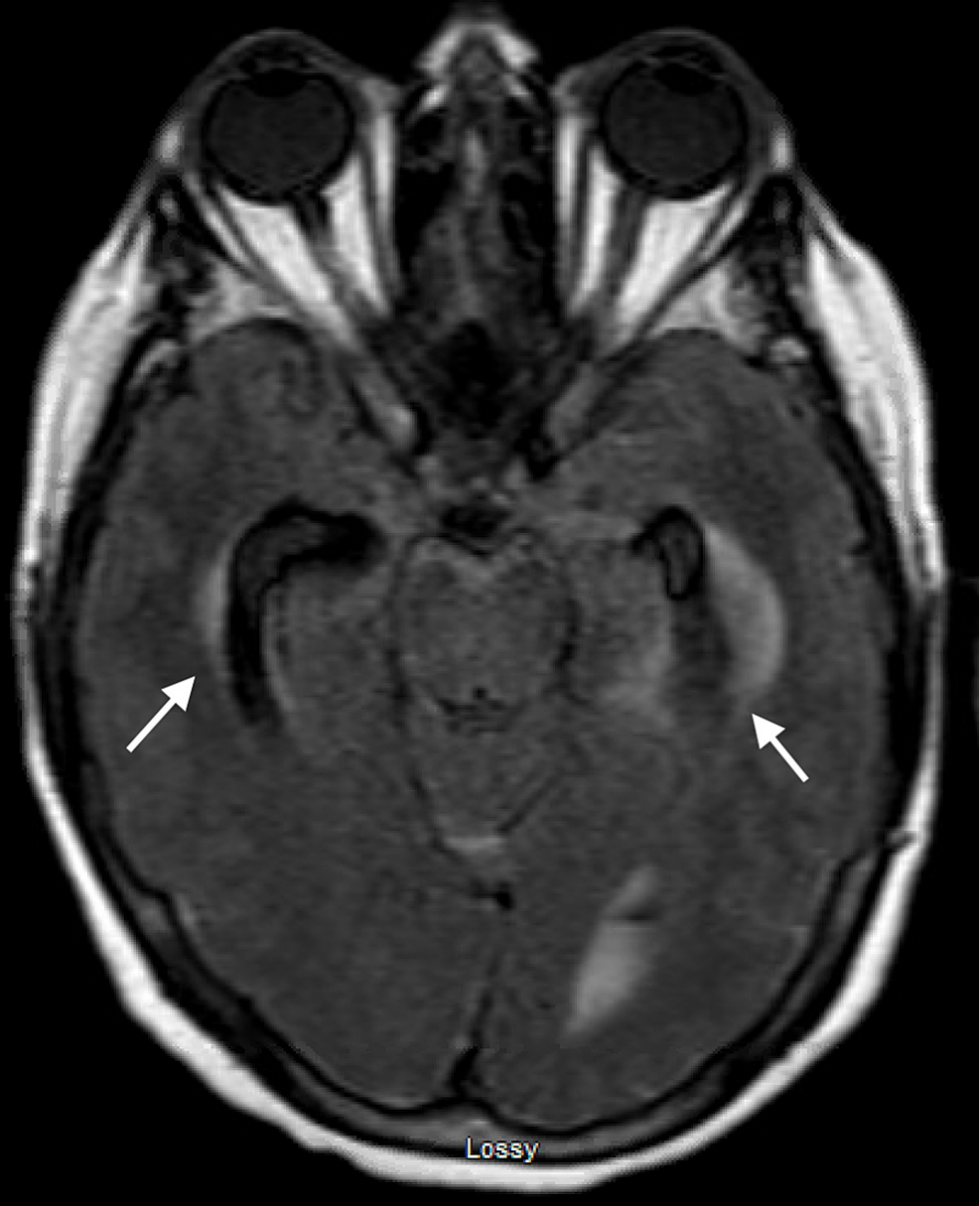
MRI brain FLAIR sequence demonstrating enlargement of the bilateral temporal horns of the ventricles with associated transependymal edema. MRI, magnetic resonance imaging; FLAIR, fluid-attenuated inversion recovery.
